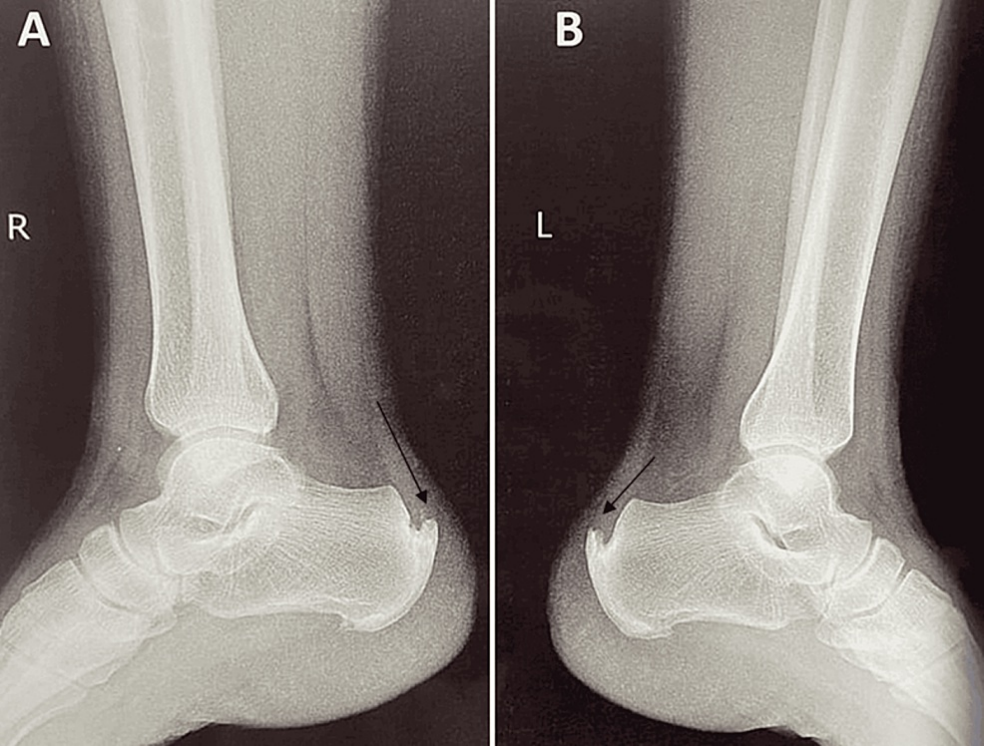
Radiological investigation shows bilateral heel spurs A: X-ray of the right heel; B: X-ray of the left heel
